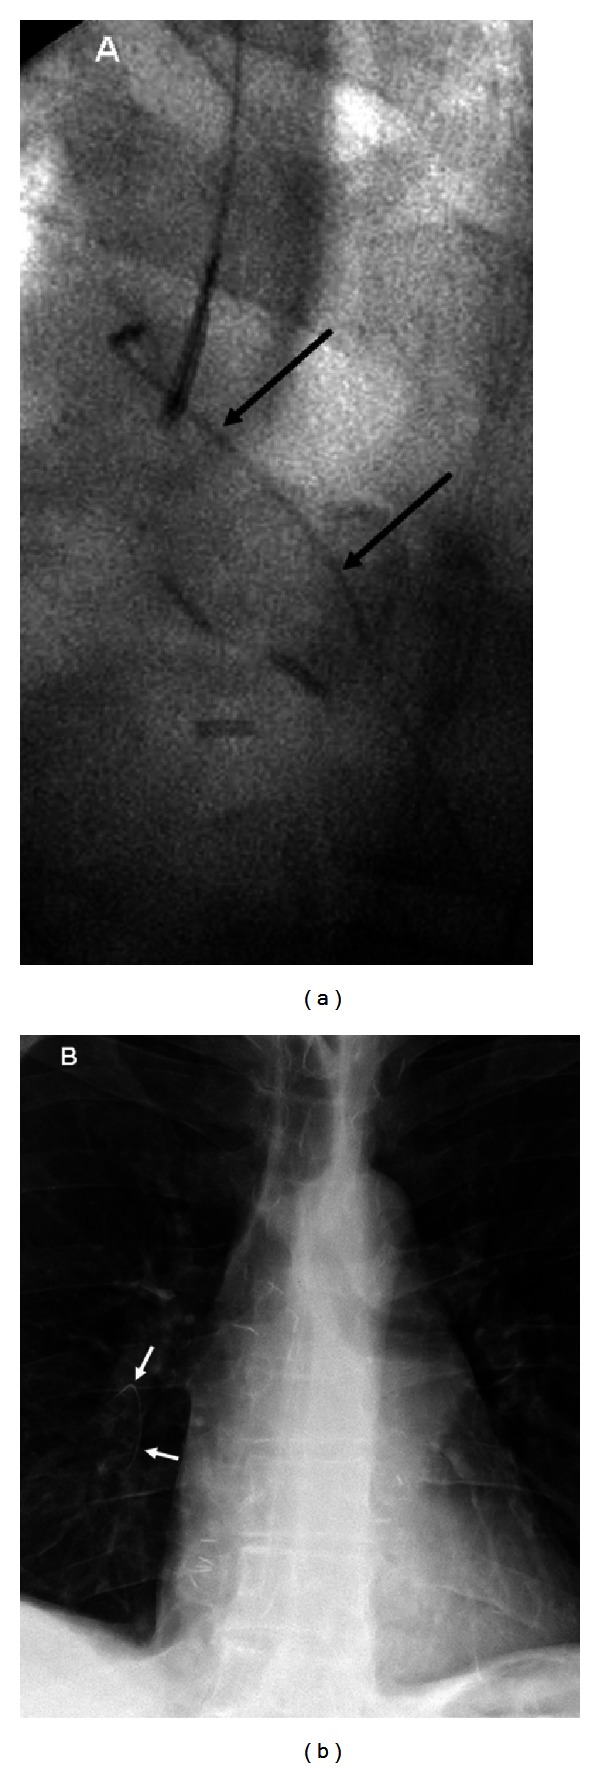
Fluoroscopic image (a) shows the foreign body successfully grasped with a 5-French goose neck snare. Chest radiograph (b) shows the embolized proximal fragment *arrows *within the right lower lobe pulmonary artery.
